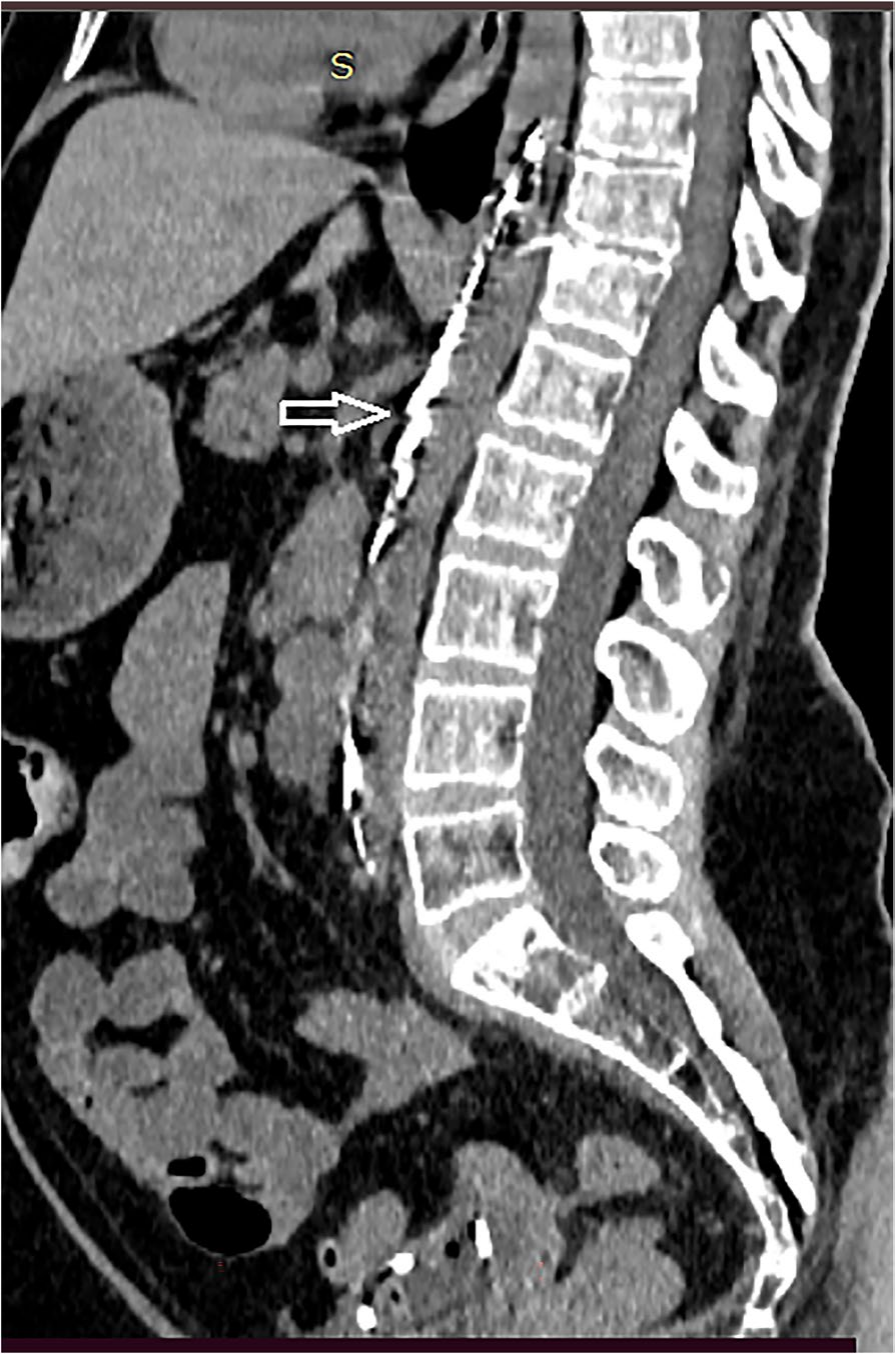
Computed tomography of the abdomen and pelvis shows the position of the ureteral stent in the abdominal aorta (arrow), sagittal plane
